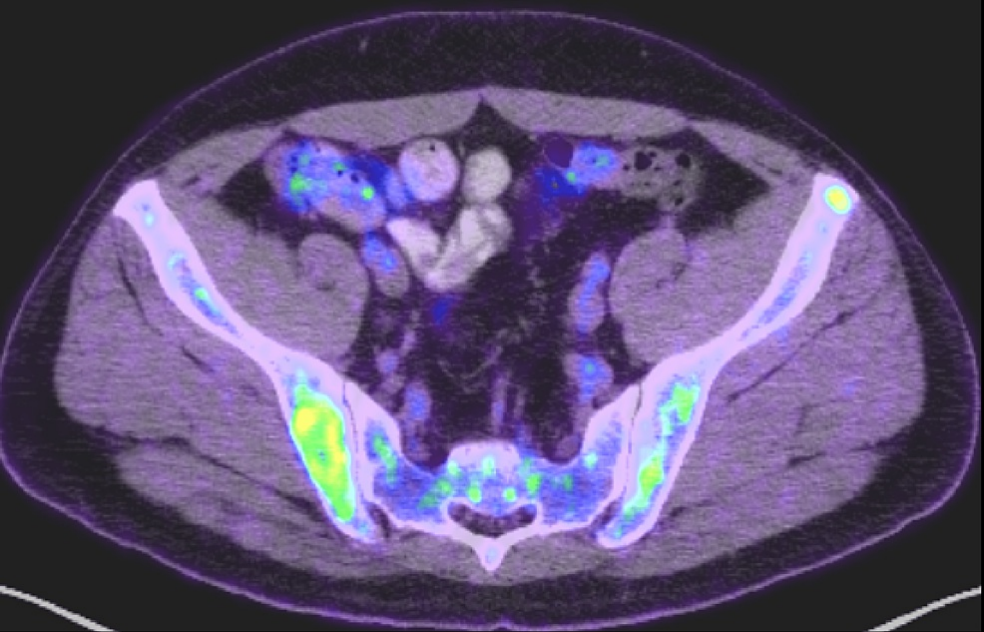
Whole-body FDG-PET/CT shows increased metabolic activity in multiple lytic skeletal lesions.
